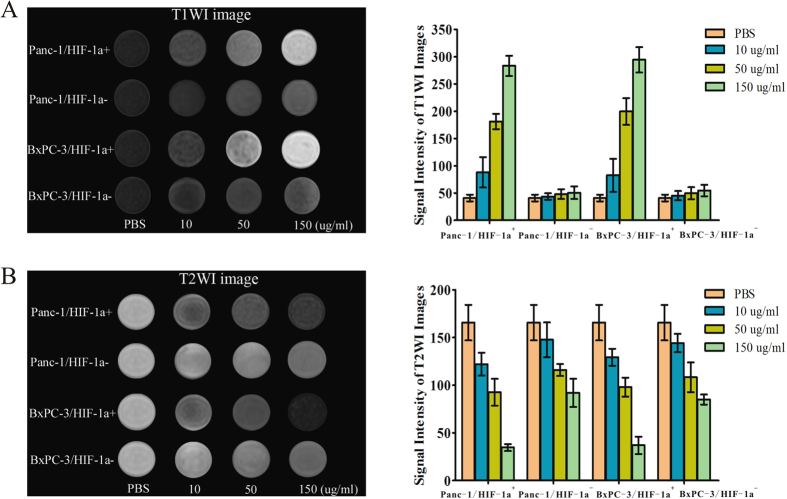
In vitro* targeted MR imaging of cancer cells. (A) T1WI MR images and signal intensity analysis for T1WI MR images of Panc-1/HIF-1α^+^, Panc-1/HIF-1α^−^, BxPC-3/HIF-1α^+^ and BxPC-3/HIF-1α^−^ incubated with varied concentrations of D-Fe_3_O_4_@PMn NPs (0, 10, 50, and 150 μg/mL) for 24 h on 3.0 T MR system. (B) T2WI MR images and signal intensity analysis for T2WI MR images of Panc-1/HIF-1α^+^, Panc-1/HIF-1α^−^, BxPC-3/HIF-1α^+^ and BxPC-3/HIF-1α^−^ incubated with various concentrations of D-Fe_3_O_4_@PMn NPs (0, 10, 50, and 150 μg/mL) for 24 h on 3.0 T MR system. (HIF-1α^+^:cancer cells express high level of HIF-1α, HIF-1α^−^: cancer cells are transfected with HIF-1α siRNA and express a low level of HIF-1α).
